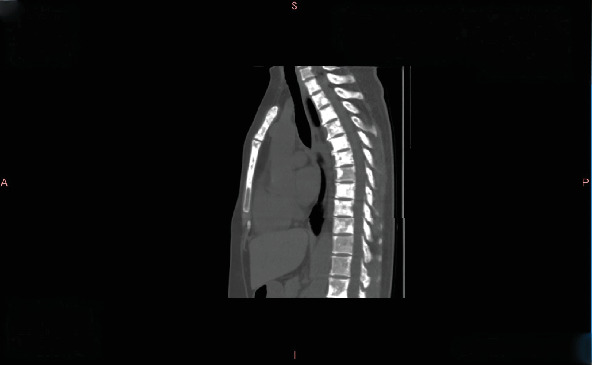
Chest CT in February 2024: multiple bone metastases similar to previous findings.
